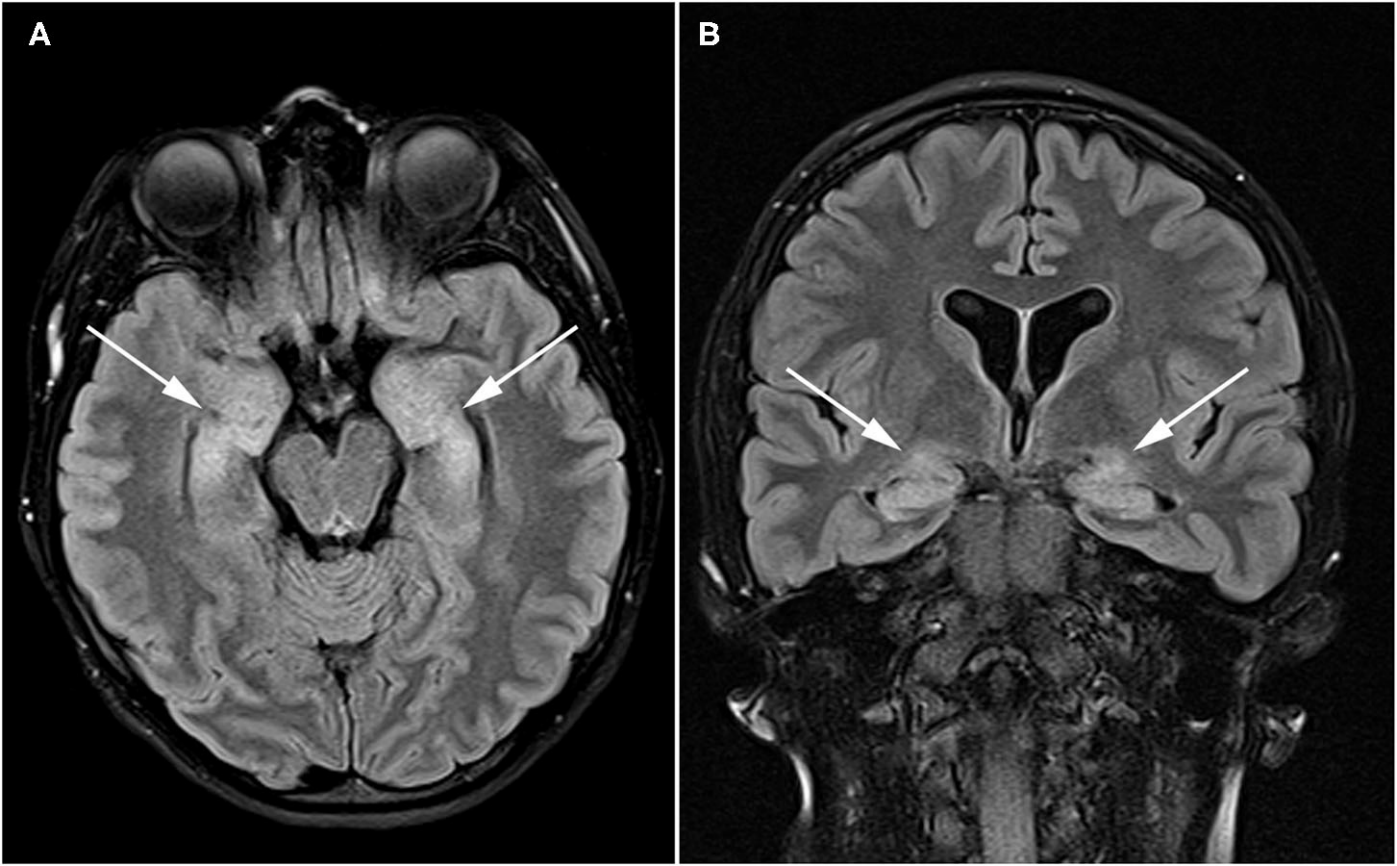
Patient with autoimmune enteric nervous system dysfunction and limbic encephalitis. Axial (A) and coronal (B) FLAIR images demonstrate high signal throughout the bilateral hippocampi and amygdala (arrows) compatible with limbic encephalitis.
